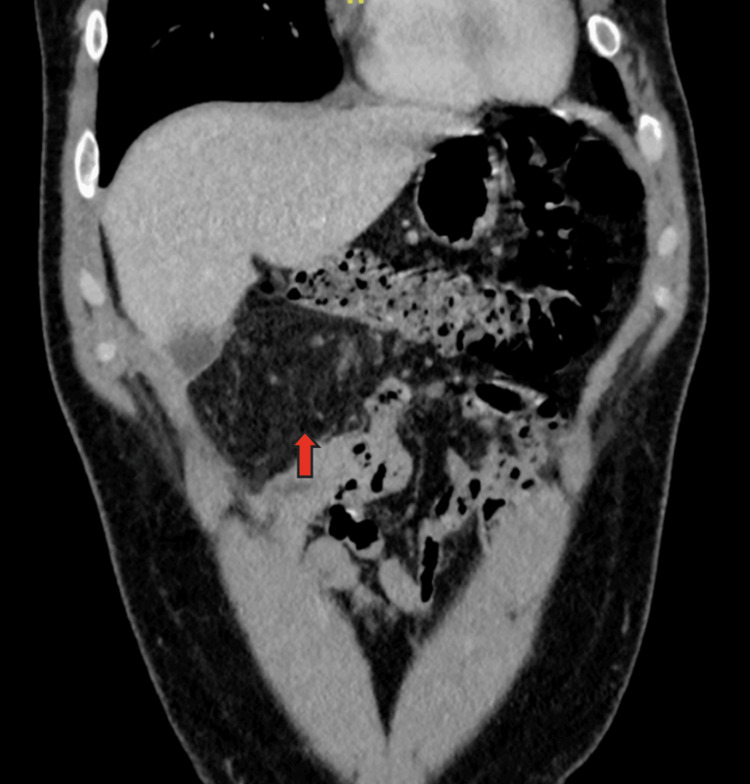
Contrast-enhanced CT of the abdomen and pelvis, coronal view A diffuse and ill-defined increase in the density of the omental adipose tissue in the right flank can be observed (arrow), suggestive of omental infarction.
